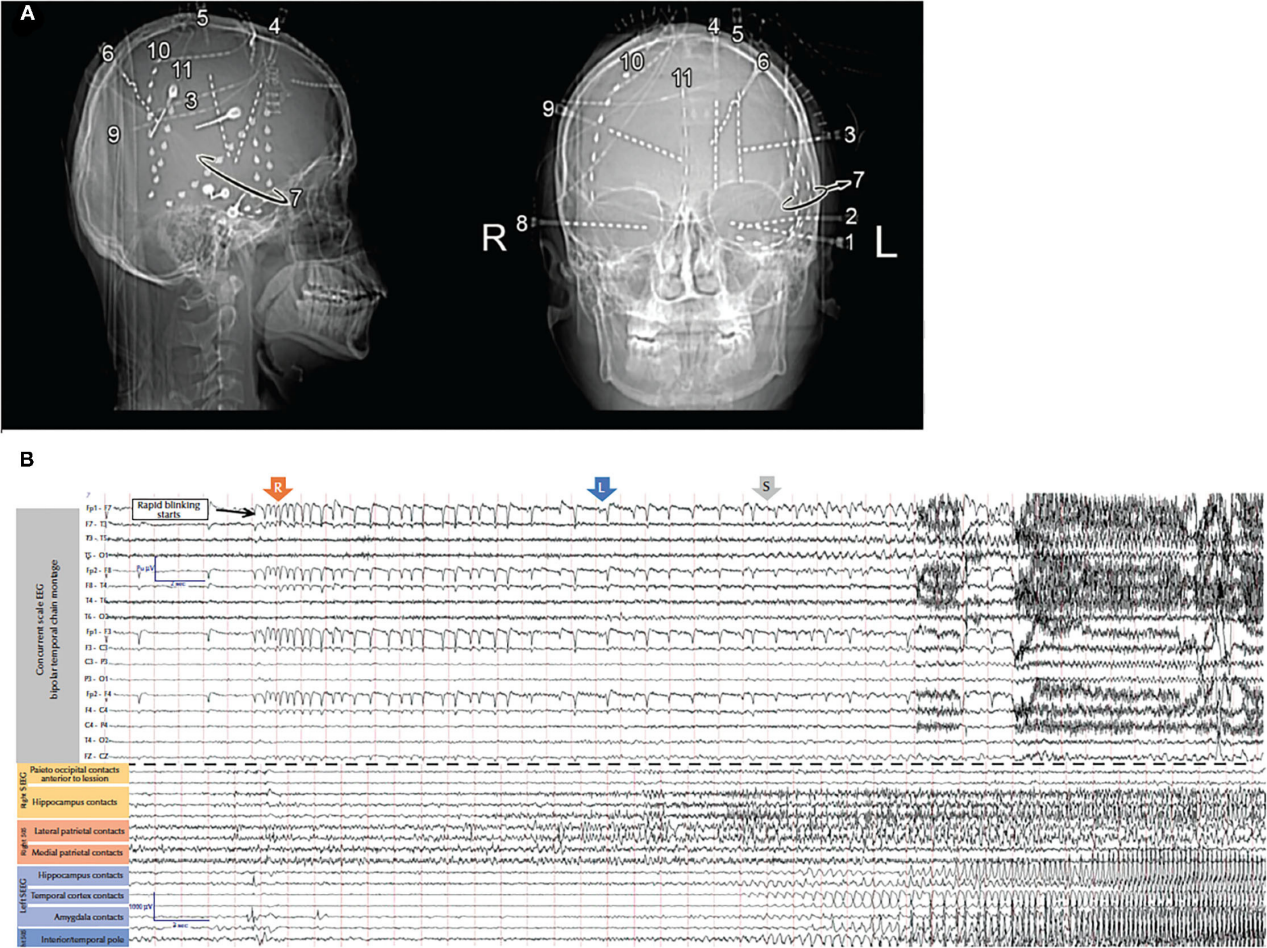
(A) X-rays showing the locations of the intracranial electrodes. (B) Scalp (top) and intracranial (bottom) electrodes in the amygdala, hippocampus, insula, and posterior cingulate gyrus in addition to the strips sampling right parietal lesion. The EEG shows onset around the right parietal lesion with secondary propagation to the contralateral temporal lobe [adapted from (8)].
